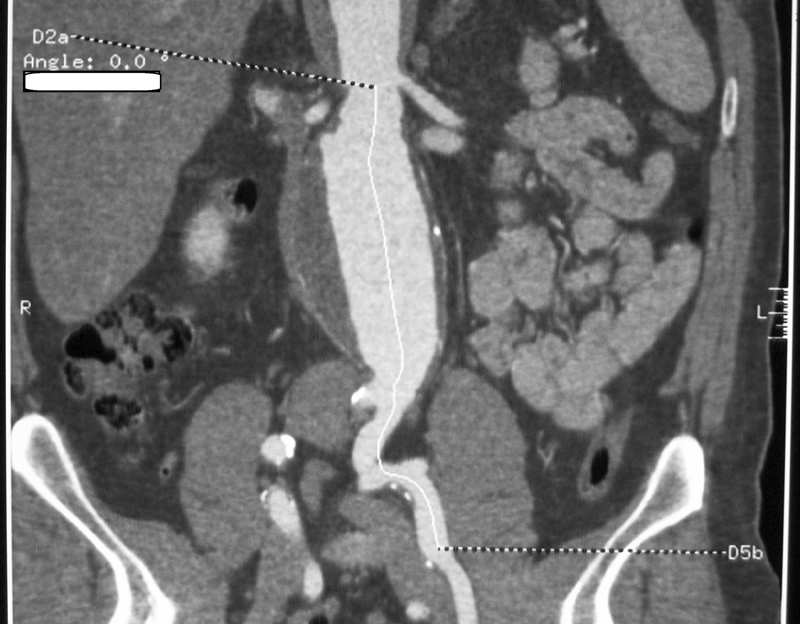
Computed tomography angiography indicates the 1 cm in length reverse taper infrarenal neck of the abdominal aortic aneurysm. No excessive calcium was shown.
